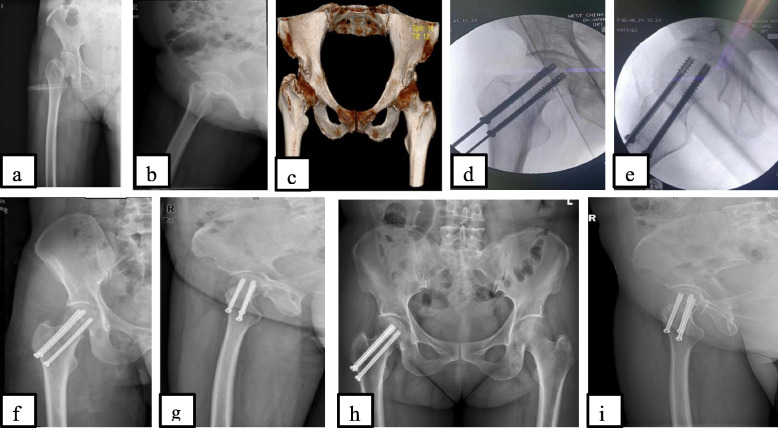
A 48 years old female patient who sustained a femoral neck fracture garden III after a fall, managed operatively with cannulated cancellous screw 24 h after injury. Preoperative X-ray anteroposterior view (a), lateral view (b) and 3D CT scan (c); intraoperative fluoroscopic images anteroposterior view (d), lateral view (e); immediate postoperative X-ray anteroposterior view (f) and lateral view (g); postoperative 12 months radiographs anteroposterior view (h) and lateral view (i)
